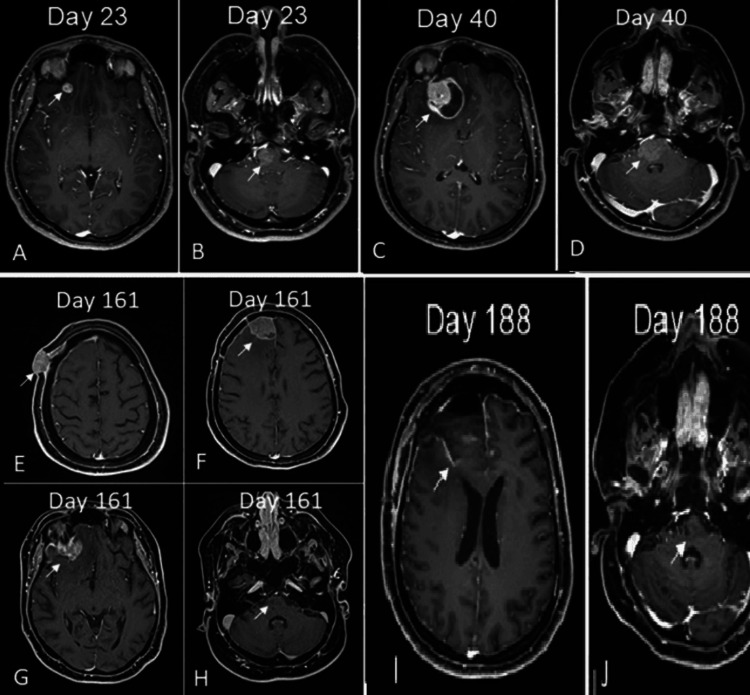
Follow-up MRIs of the patient (A,B) The lesion at the anterior midline and anterior right side of the medulla oblongata-bulbus junction and the basal area of the right frontal lobe, showing patchy gadolinium enhancement. (C,D) One month later, the lesion in the frontal lobe was growing in an expansile pattern. (E-G) The recurrent lesions at the basal area of the right frontal lobe and invasion to the skin area, which show patchy gadolinium enhancement. (H) The lesion at the anterior midline and anterior right side of the medulla oblongata-bulbus junction, which shows a significant decrease in gadolinium enhancement. (I) The postoperative images of the recurrent lesion at the basal area of the right frontal lobe. (J) The lesion at the anterior midline and anterior right side of the medulla oblongata-bulbus junction shows a significant decrease in gadolinium enhancement MRI: magnetic resonance imaging
